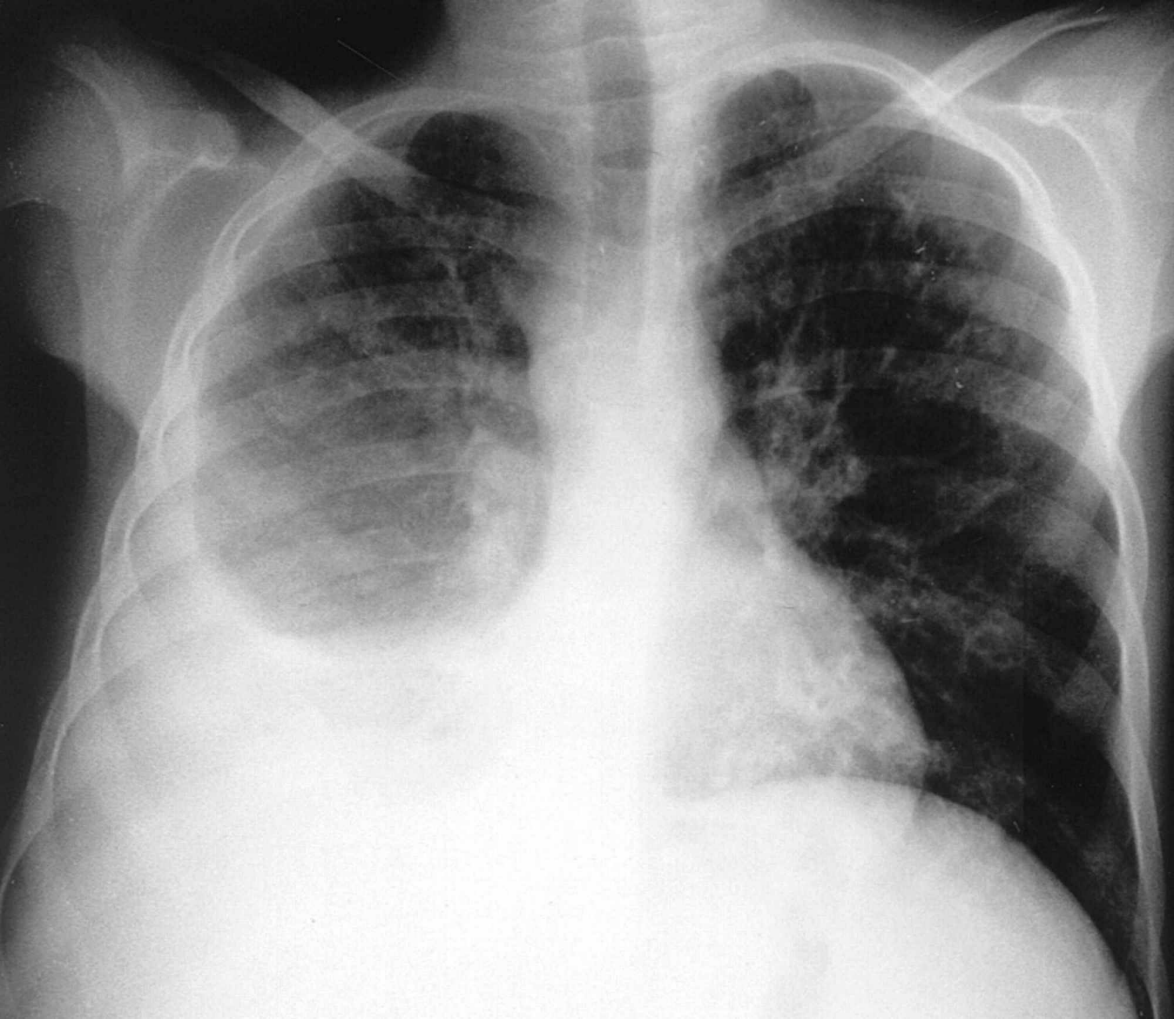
Chest radiography demonstrating right-sided pleural effusion.
